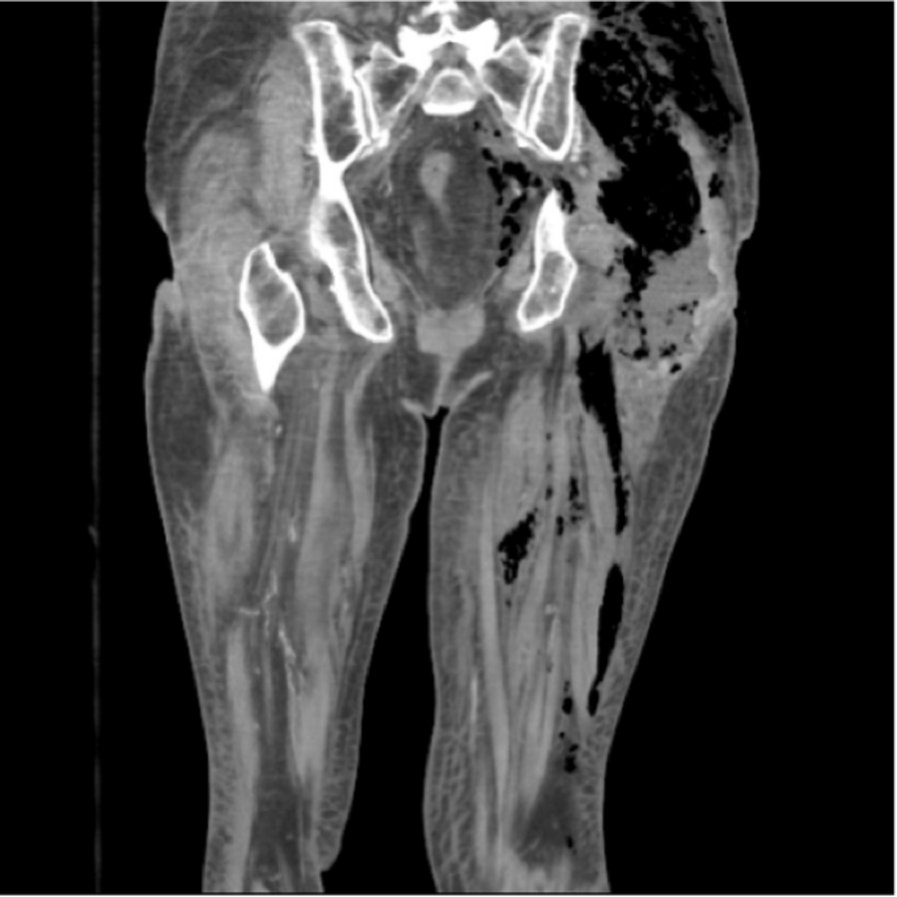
Frontal view of CT Pelvis & Lower extremities demonstrating subcutaneous gas tracking along fascial planes of left leg and into hemipelvis Courtesy of https://emrems.com/2013/04/06/necrotizing-fasciitis-2/
